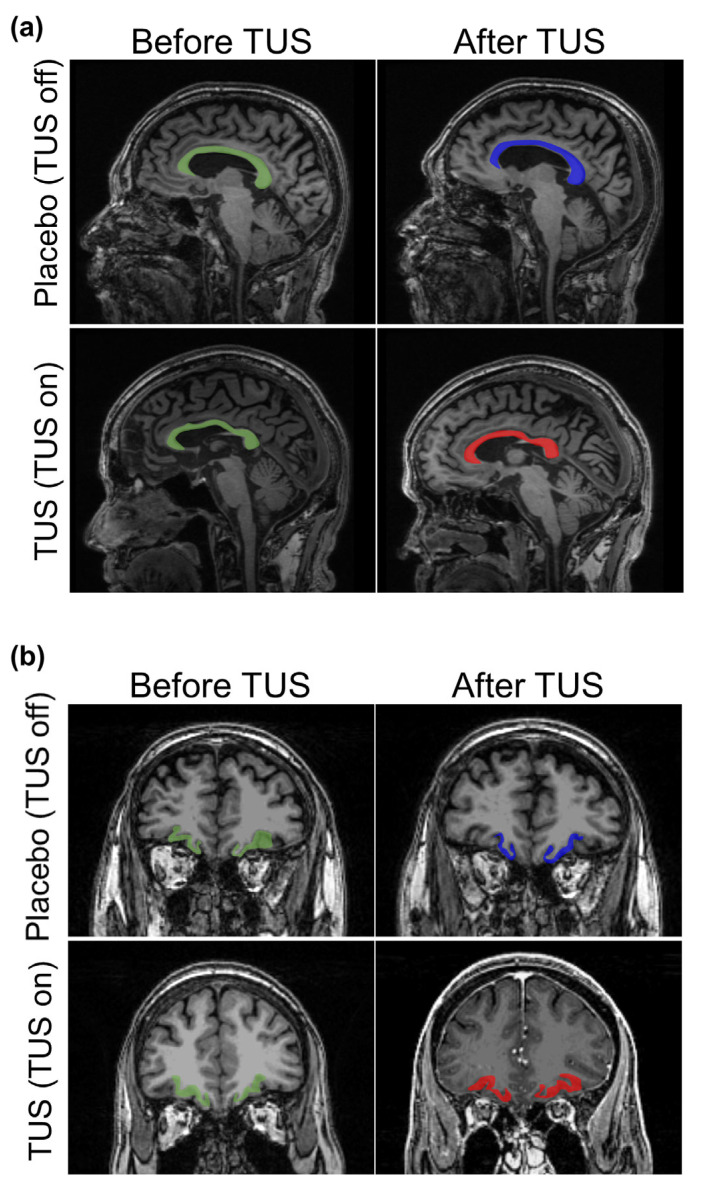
Representative T1-weighted magnetic resonance imaging (MRI) scan of patients with Alzheimer’s disease. (a) The corpus callosum before transcranial ultrasound stimulation (TUS) is highlighted in green. After TUS, the corpus callosum of the placebo group is highlighted in blue, while the corpus callosum of the TUS group is highlighted in red. First row represents the Placebo group. Second row represents the TUS group. For the detailed structure and function of the corpus callosum, please refer to Figure S2 in the supplementary file. (b) The lateral orbitofrontal cortex before TUS is highlighted in green. After TUS, the lateral orbitofrontal cortex of the placebo group after TUS is highlighted in blue, while the lateral orbitofrontal cortex of the TUS group is highlighted in red. First row represents Placebo group. Second row represents TUS group.
